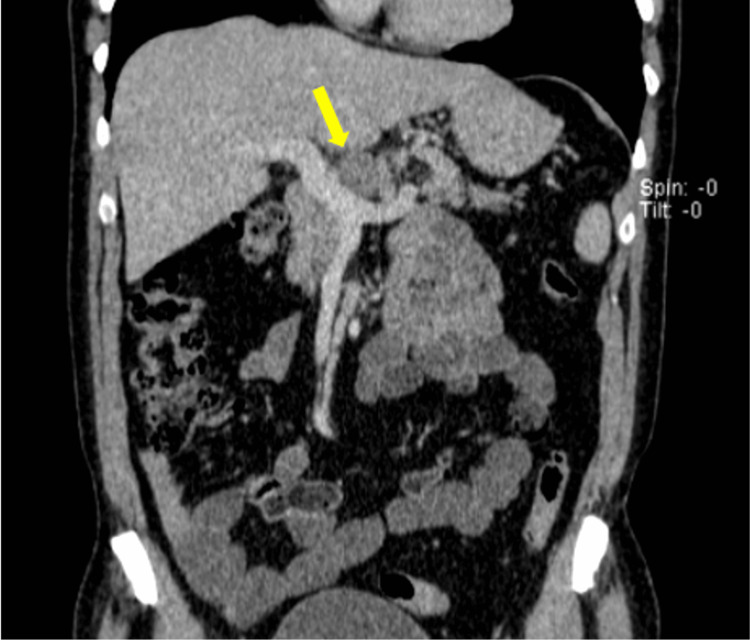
Coronal contrast-enhanced CT following chemotherapy showing a marked reduction in size of the primary pancreatic lesion (yellow arrow), indicating excellent treatment response.
